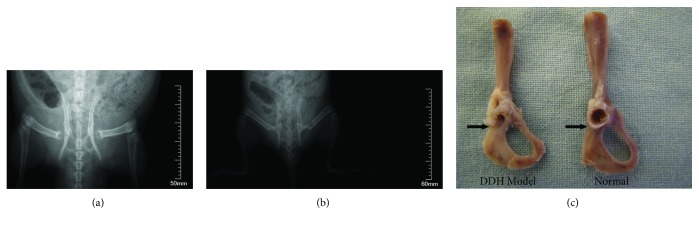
Anteroposterior pelvic radiographs and acetabular tissue of DDH model hip and normal hip. (a) Dislocation in both right and left hip of DDH model. (b) Anteroposterior pelvic radiographs of normal hip. (c) A dysplastic true acetabulum of DDH model hip (left) and normal acetabulum of normal hip (right).
